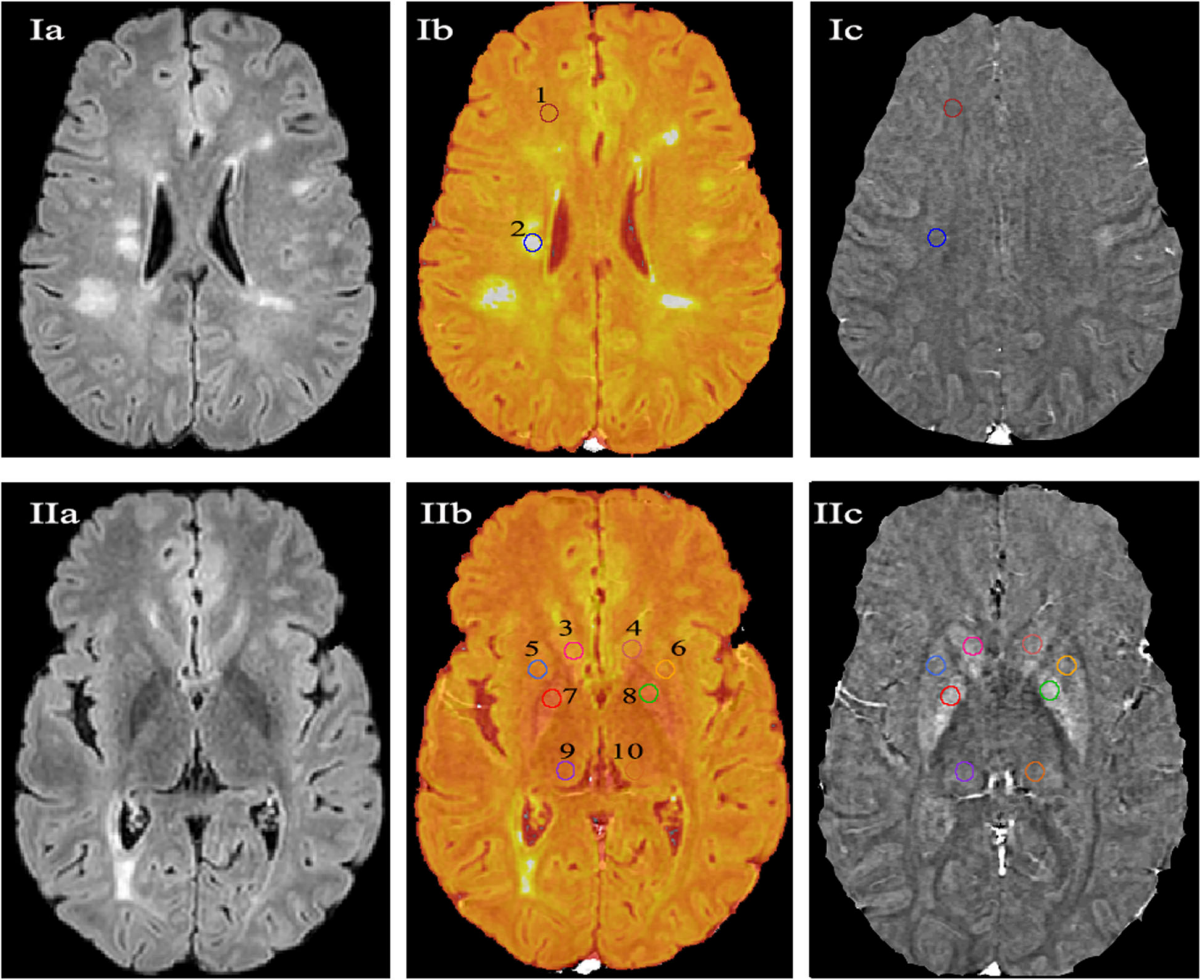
Measurement of regional iron in a 25-year-old man with MS. Images Ia, Ib, and Ic represent the steps of measuring iron concentrations in the normal appearing white matter (region of interest [ROI] 1) and multiple sclerosis lesions (ROI 2). Ia is an axial three-dimensional fluid-attenuation inversion recovery (3D FLAIR) film demonstrating the MS lesions. Ib is the 3D FLAIR image overlayed onto the quantitative susceptibility mapping, and Ic is the quantitative susceptibility map. Similar steps are demonstrated in IIa, IIb, and IIc to measure iron concentrations in the deep grey matter: caudate (ROIs 3 and 4), putamen (ROIs 5 and 6), globus pallidus (ROIs 7 and 8), and thalami (ROIs 9 and 10).
